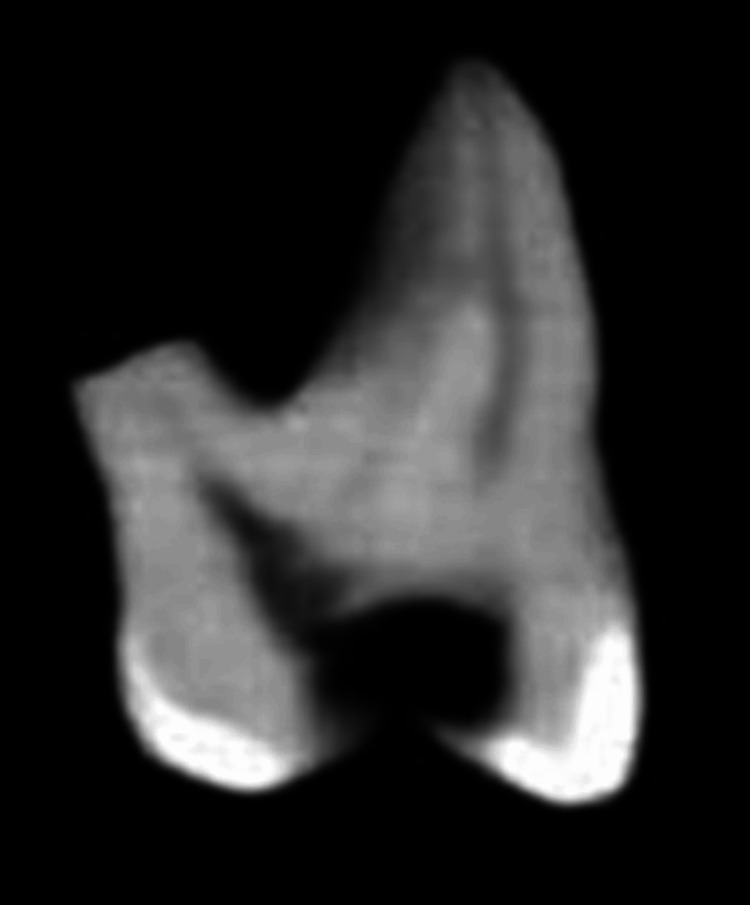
Cone-beam computed tomography (CBCT) scan of the tooth in the study
